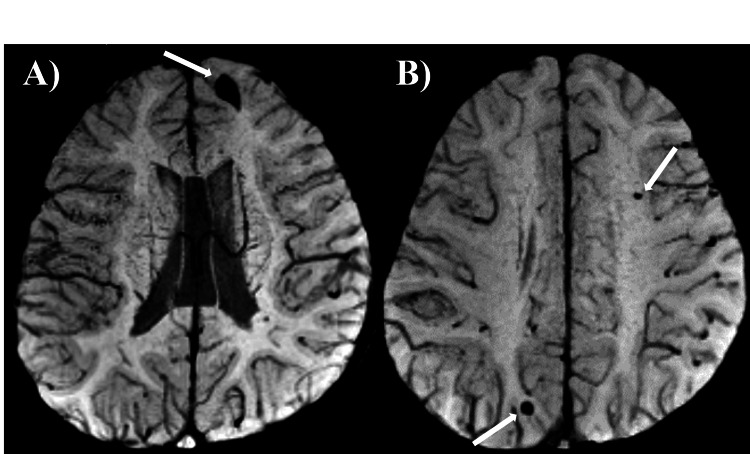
Brain magnetic resonance imaging at six years of age (A, B) Multiple small hypointense lesions in the left frontal lobe (A), left parietal lobe and right occipital lobe (B) were detected on T2*-weighted magnetic resonance imaging.
